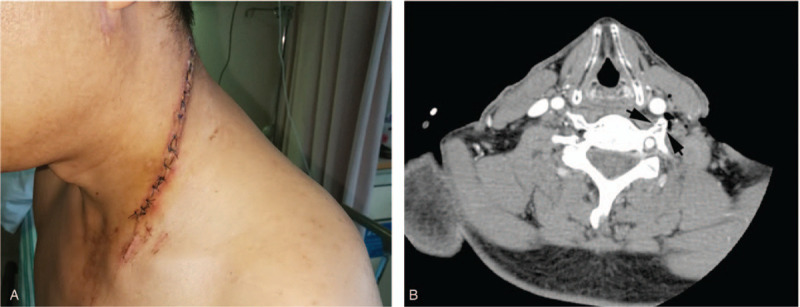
The left neck wound (A, day 4), and neck CT scan on admission showed a fracture of the transverse process of C5 (black arrows, B).
